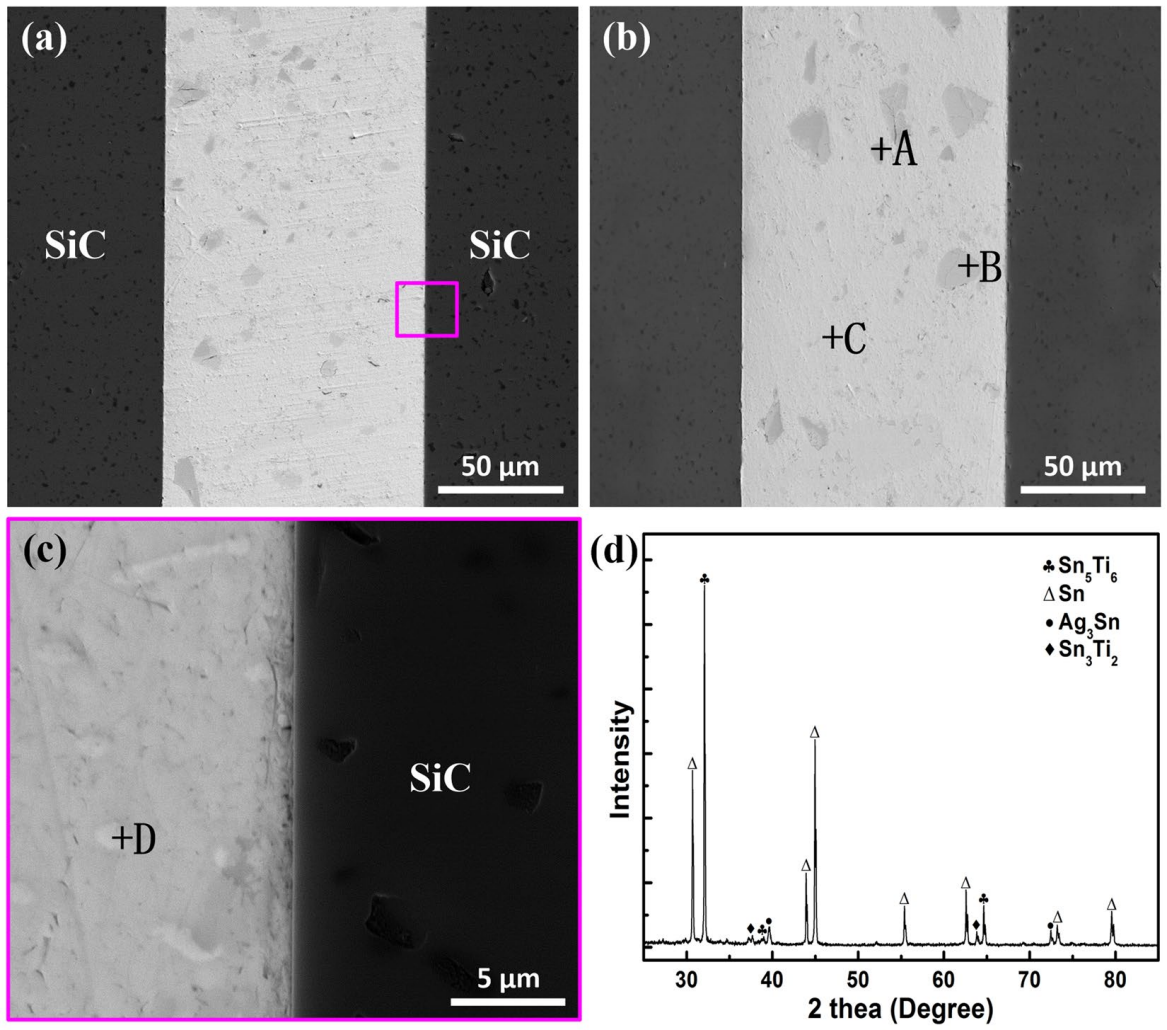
Typical SEM images of the as-formed SiC joints soldered under the ultrasonic action for (a) 0.1 s and (b) 1 s; (c) the corresponding magnification views as marked in (a); (d) MF-XRD patterns of the bond layer in (a).
